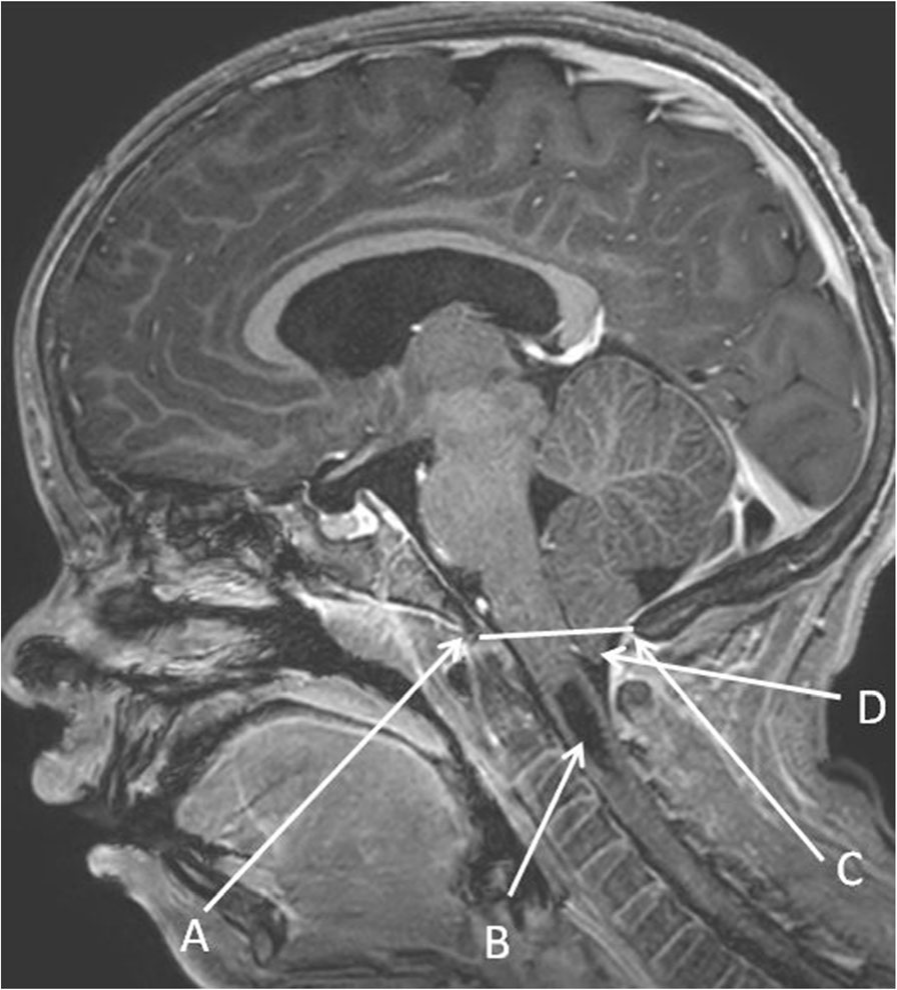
Brain MRI at 9 years 10 months, structures as labeled. Depicted is a Chiari I malformation with a syrinx at C1-C4. a Basion, b Syrinx, c Opisthion, d Low lying cerebellar tonsil
